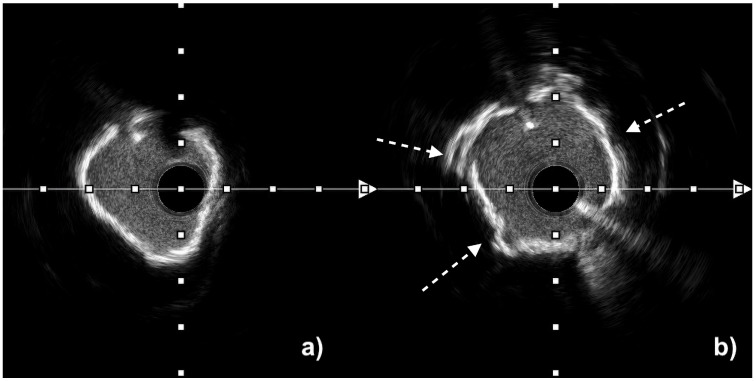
Intravascular ultrasound (IVUS) images of a severely calcified coronary lesion with circumferential (360°) calcification before (a) and after (b) intravascular lithotripsy (IVL) using the Shockwave C2+ catheter (Shockwave Medical, USA), demonstrating an increase in minimal lumen area and multiple calcium fractures (indicated by white arrows).
